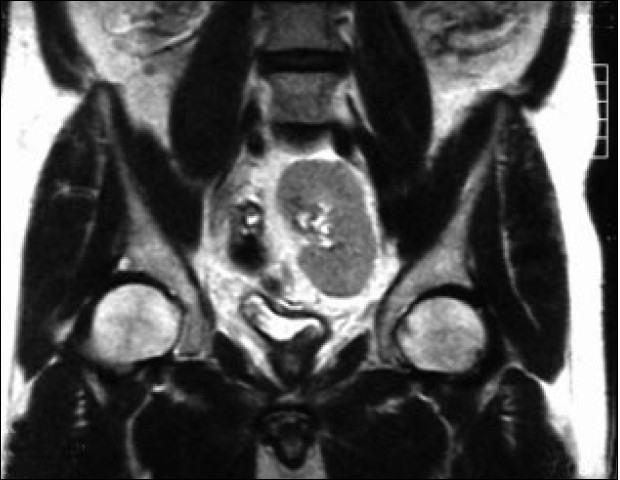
Postoperative MRI (Coronal *T*_2_-weighted image) showing a pelvic kidney with a normal nephrogram and the surrounding fatty tissue
